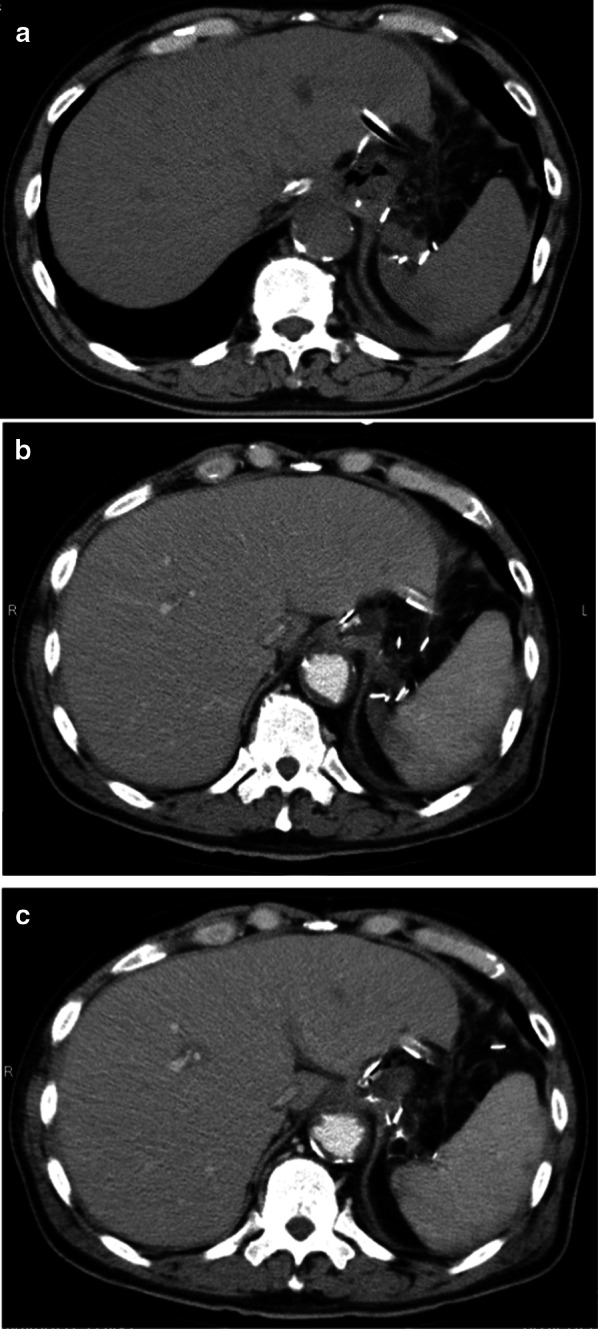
a Computed tomography (CT) images of the anastomotic site on post-operative day (POD) 8. b CT image of the anastomotic site on POD 13. (C) CT image of anastomotic site on POD 24
